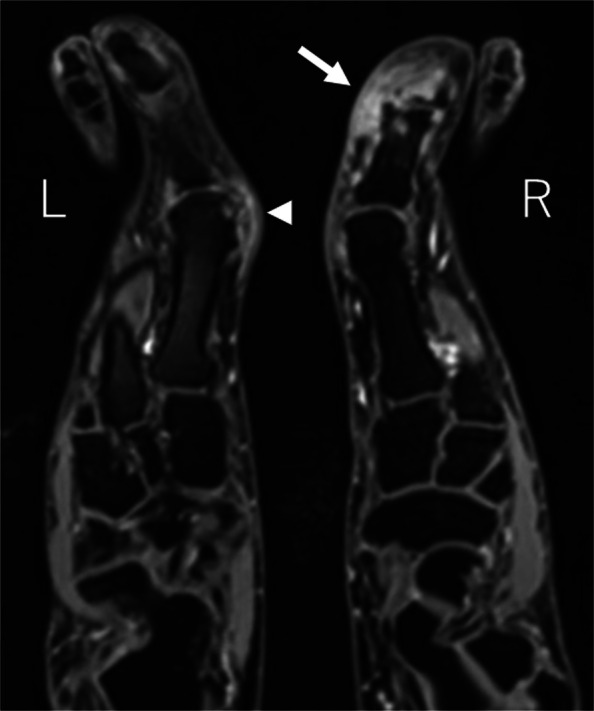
Contrast-enhanced MRI showing hypervascular tophi at the right first IP joint (arrow) and left first MTP joint (arrowhead)
